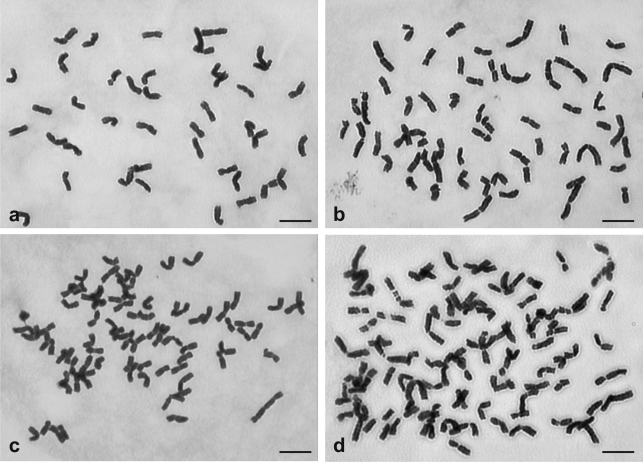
Metaphase chromosomes in four different American lines of *Bromus* subgen. *Ceratochloa*. a - hexaploid *B. willdenowii* cv. *Atom* [CT3], b - octoploid *B. marginatus* [CT20], c -duodecaploid *B. arizonicus* [CT21], d - duodecaploid from Machachi (Ecuador) [CT22]. Note the presence of longer chromosomes in CT20 and CT 22. [] indicates origin of *Bromus* samples (see Table 1). Scale bar = 5 μm.
